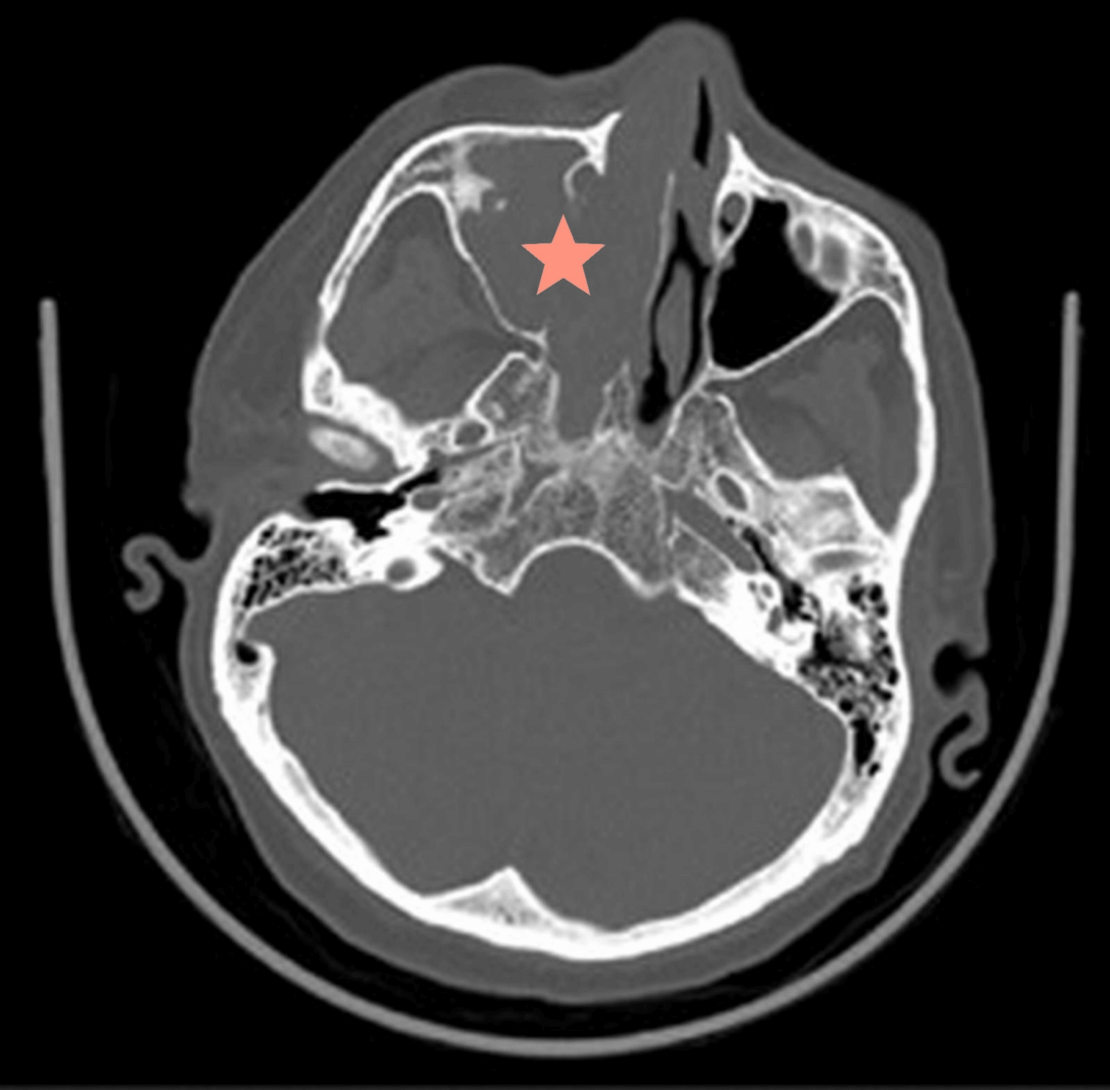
Axial brain CT bone window showing bone destruction due to aggressive nature of the tumor (star).
